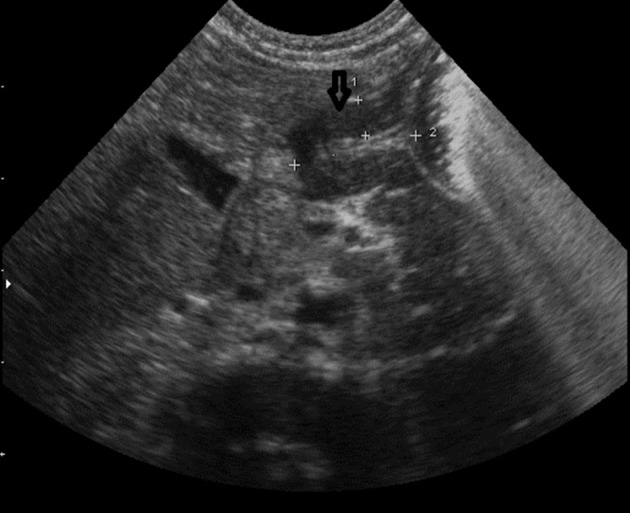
Figure 5. The classic findings of HPS demonstrated by ultrasound as abnormal muscle thickness and an elongated pyloric channel (cervix sign).
